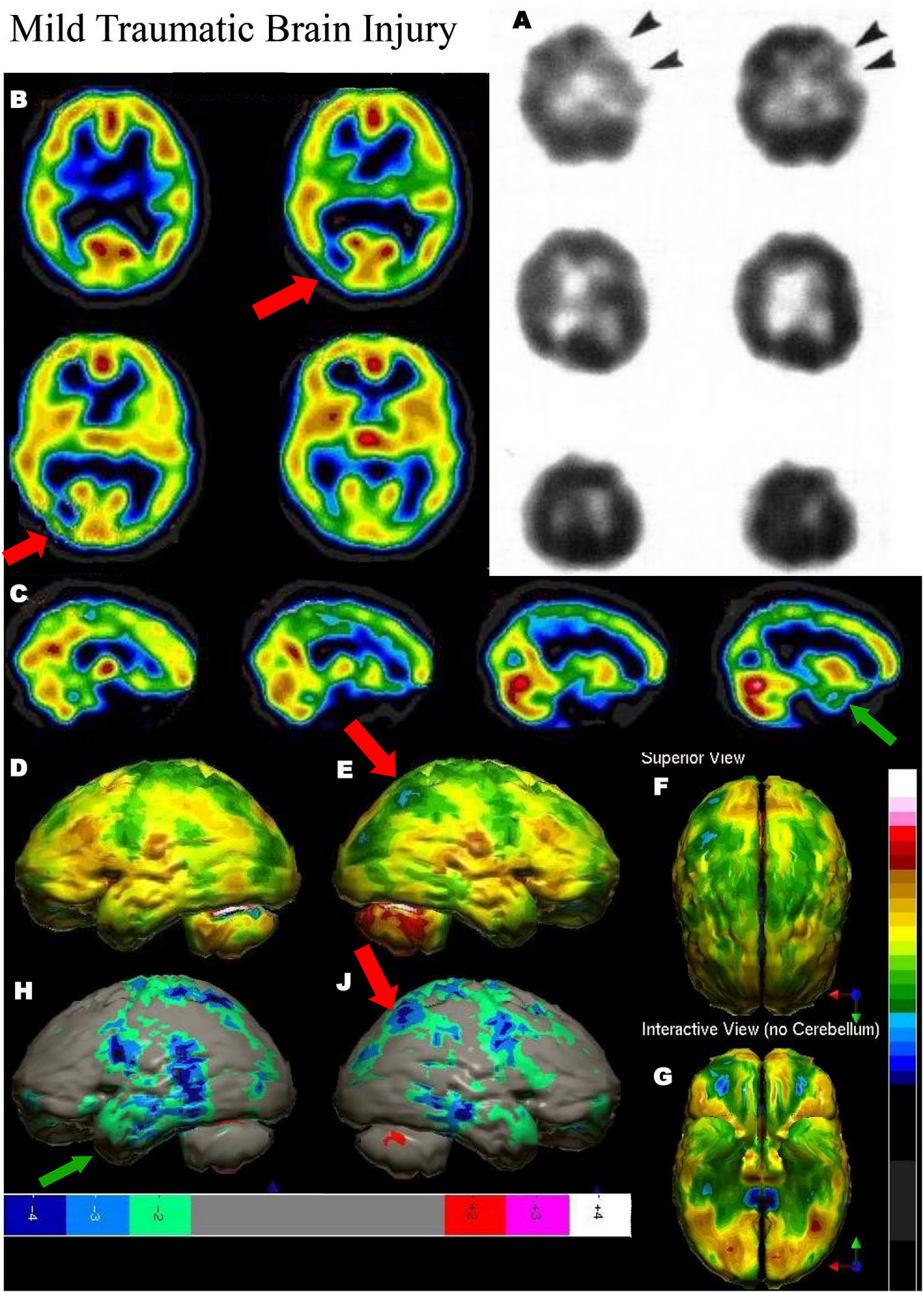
Mild TBI. (A) Example of SPECT scans cited in TTASAAN report. Anatomical details are lacking. 50-year-old male with minor head trauma after a motor vehicle accident showed decreased perfusion of the left inferior frontal and left anterior temporal lobe, whilst MRI scan was normal [The figure was originally published in JNM. © SNMMI (28)]. (B–G) A 2019 SPECT scan using a dual-headed gamma camera of an 18-year-old male who struck a tree while mountain biking and briefly lost consciousness. A modern ^99m^mTc-HMPAO perfusion SPECT scan was performed with a dual-head gamma camera. (B) Horizontal tomograms show detail of thalamus, anterior cingulate, caudate, and lentiform nuclei. A focal area of hypoperfusion can be seen in the right parietal cortex (red arrow). Color scale is the same as in Figure 1B. (C) Sagittal tomograms reveal decreased inferior frontal perfusion and decreased medial temporal perfusion bilaterally (green arrow). (D–G) 3-D representation of SPECT scan data showing left lateral (D), right lateral showing the area of hypoperfusion in the right parietal cortex (red arrow) (E), superior (F), and inferior views (G). (H–J) The patient's data is compared to a normative database using Segami Inc. Oasis software. The color scale is the same as in Figure 2D. Areas of relatively decreased perfusion are more evident, such as the area of hypoperfusion in the right parietal cortex (red arrow).
